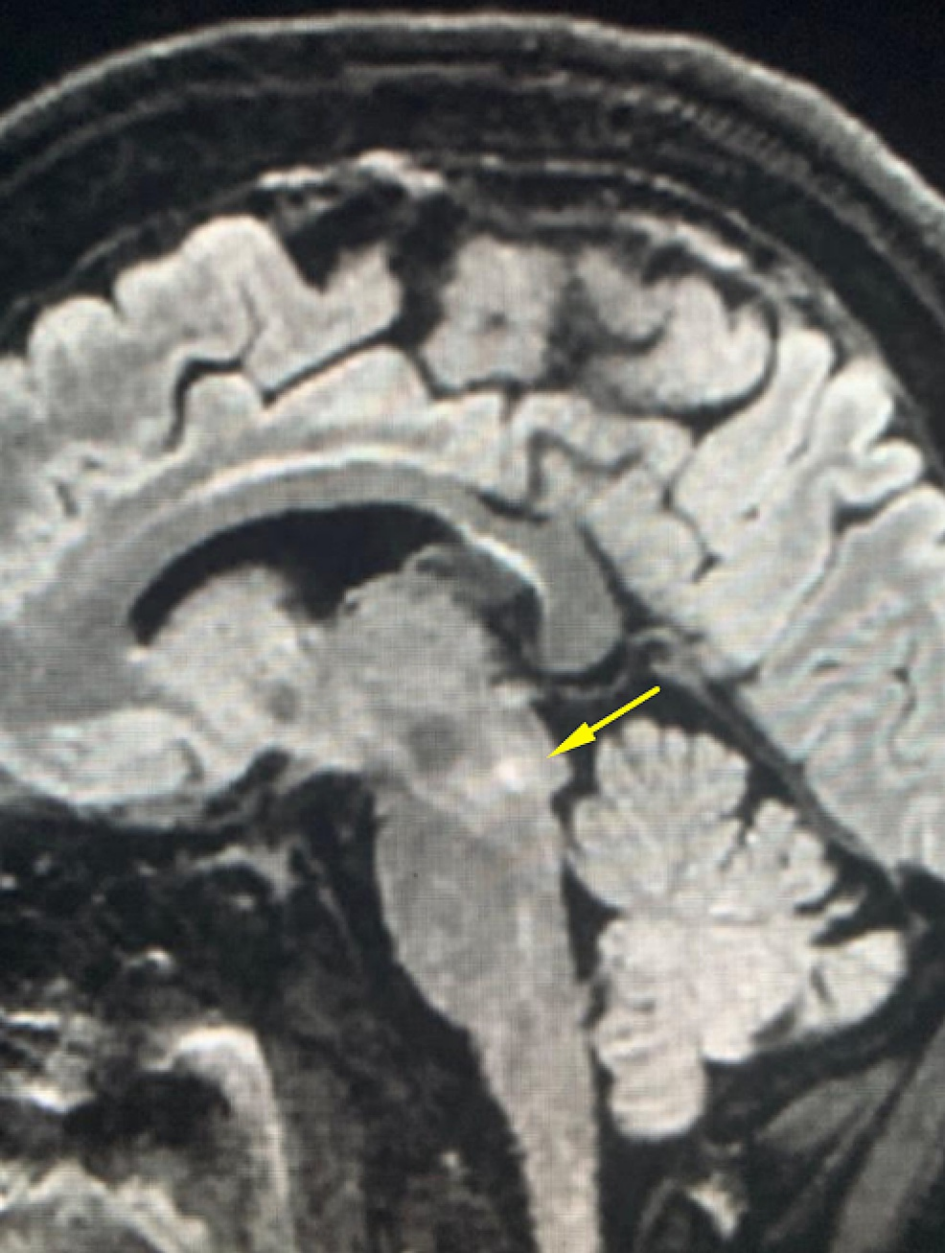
Sagittal FLAIR displaying midbrain lesion FLAIR: fluid-attenuated inversion recovery
